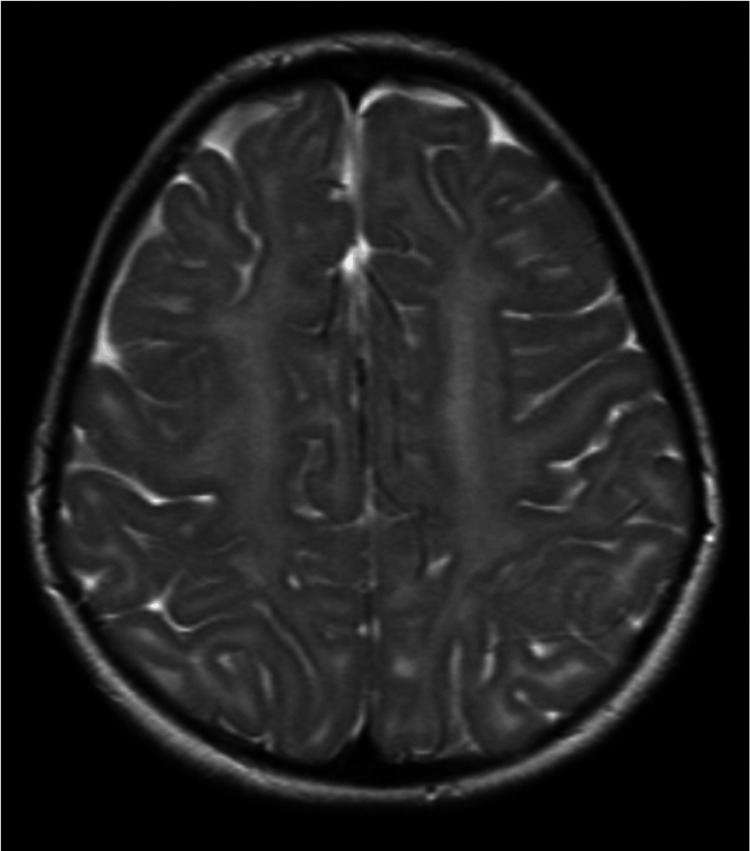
Axial T2 Weighted Imaging Follow-up axial T2-weighted image obtained at 5 years of age demonstrated persistent confluent supratentorial white matter T2 hyperintensity.
